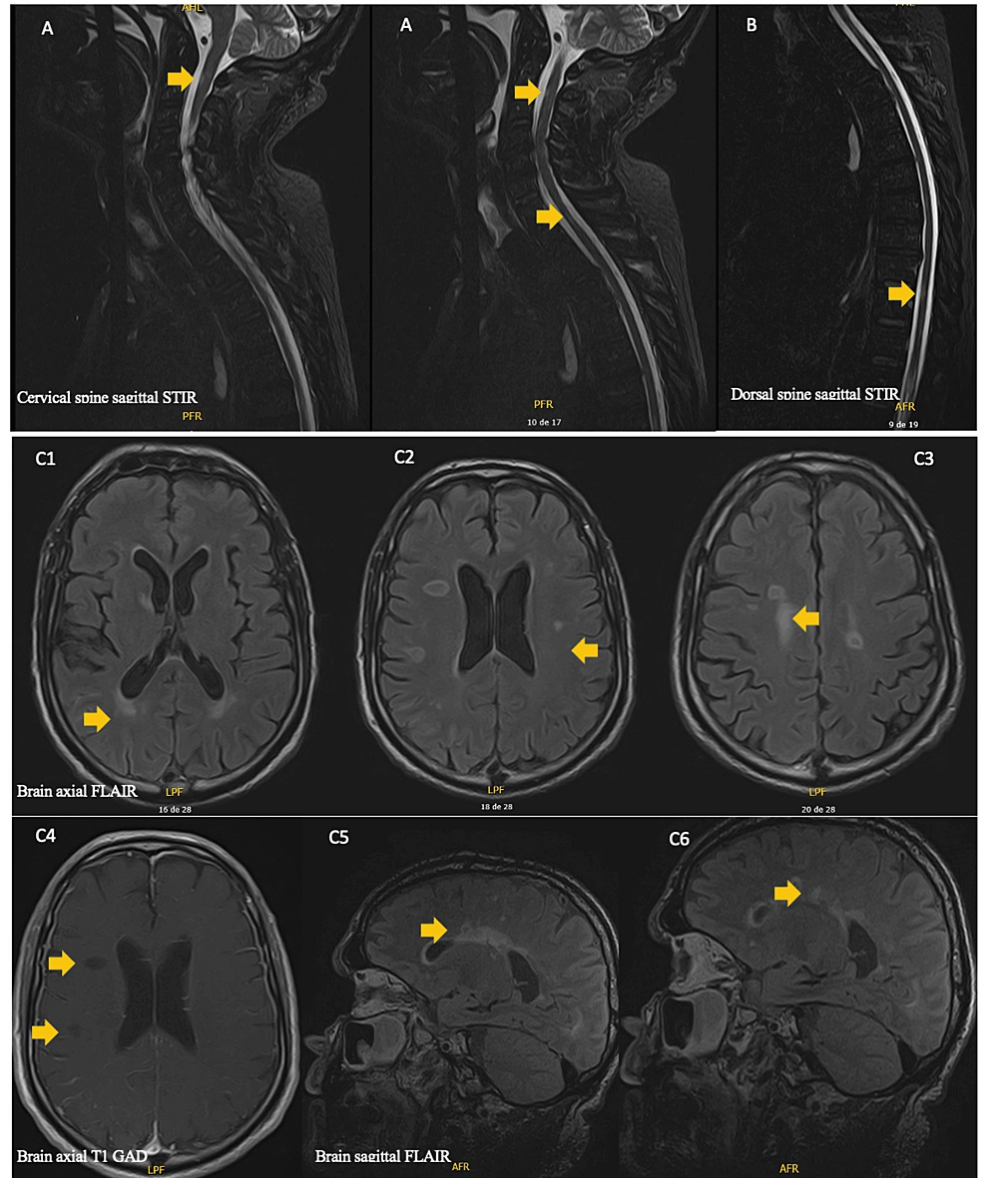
MRI showing multiple cervical (A), dorsal (B), and brain periventricular (C1), deep (C2), and juxtacortical (C3) white matter lesions without contrast-enhancing (C4). T1 black holes are indicative of a chronic stage with white matter destruction, axonal loss, and an irreversible clinical outcome (C4). Periventricular demyelinating plaques extending radially away from the body of the lateral ventricle are characteristic of multiple sclerosis (C5-6).
